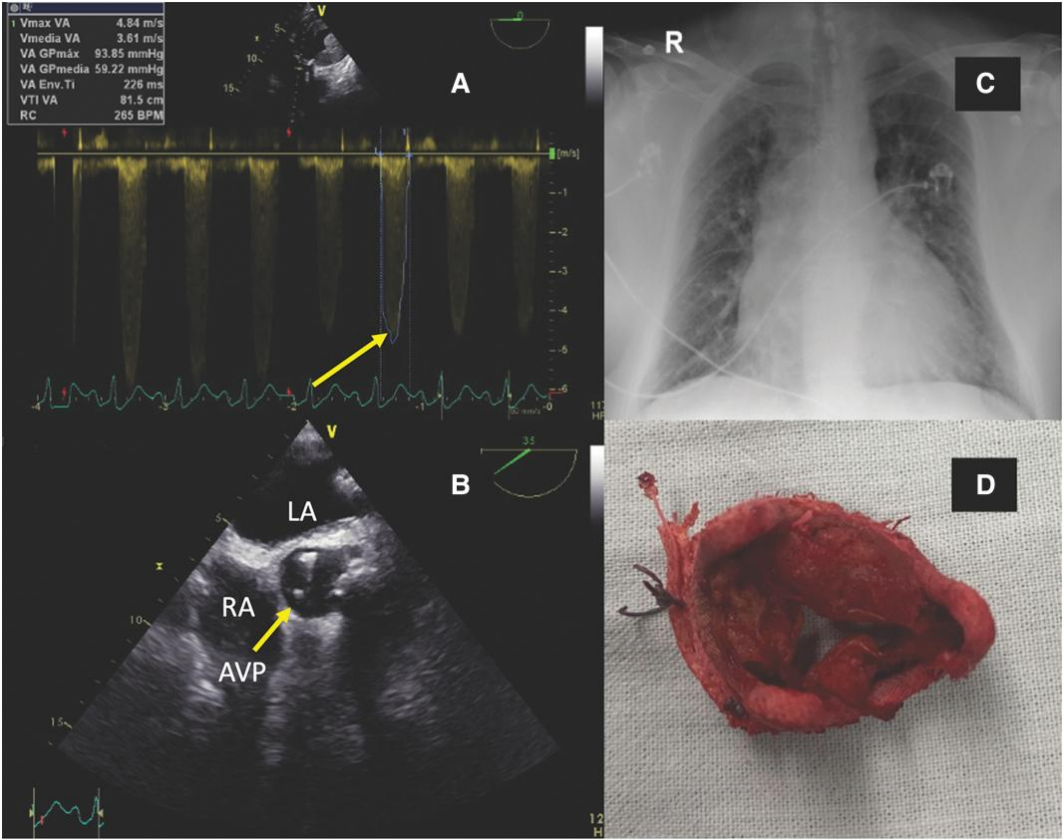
Case 1: (*A*) Continuous spectral Doppler in transoesophageal echocardiogram and deep transgastric projection of the aortic prosthesis, with high velocities and gradients (peak velocity of 4.8 ms, mean gradient of 59 mmHg, and acceleration time of 90 ms). (*B*) Short-axis transoesophageal echocardiogram in mid-oesophagus at the level of the aortic valve prosthesis. (*C*) Bilateral alveolar opacities. (*D*) Dysfunctional bioprosthetic aortic valve causing cardiogenic shock. AVP, aortic valve prosthesis.
